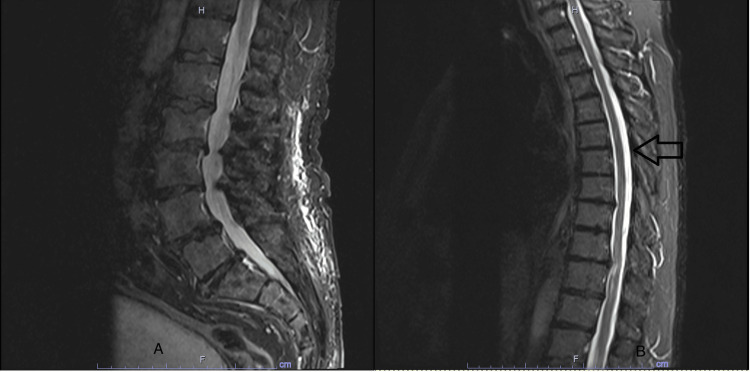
MRI of the thoracic and lumbar spine. (A) MRI of the lumbar spine and (B) MRI of the thoracic spine showing improvement in the epidural hematoma (arrows).
